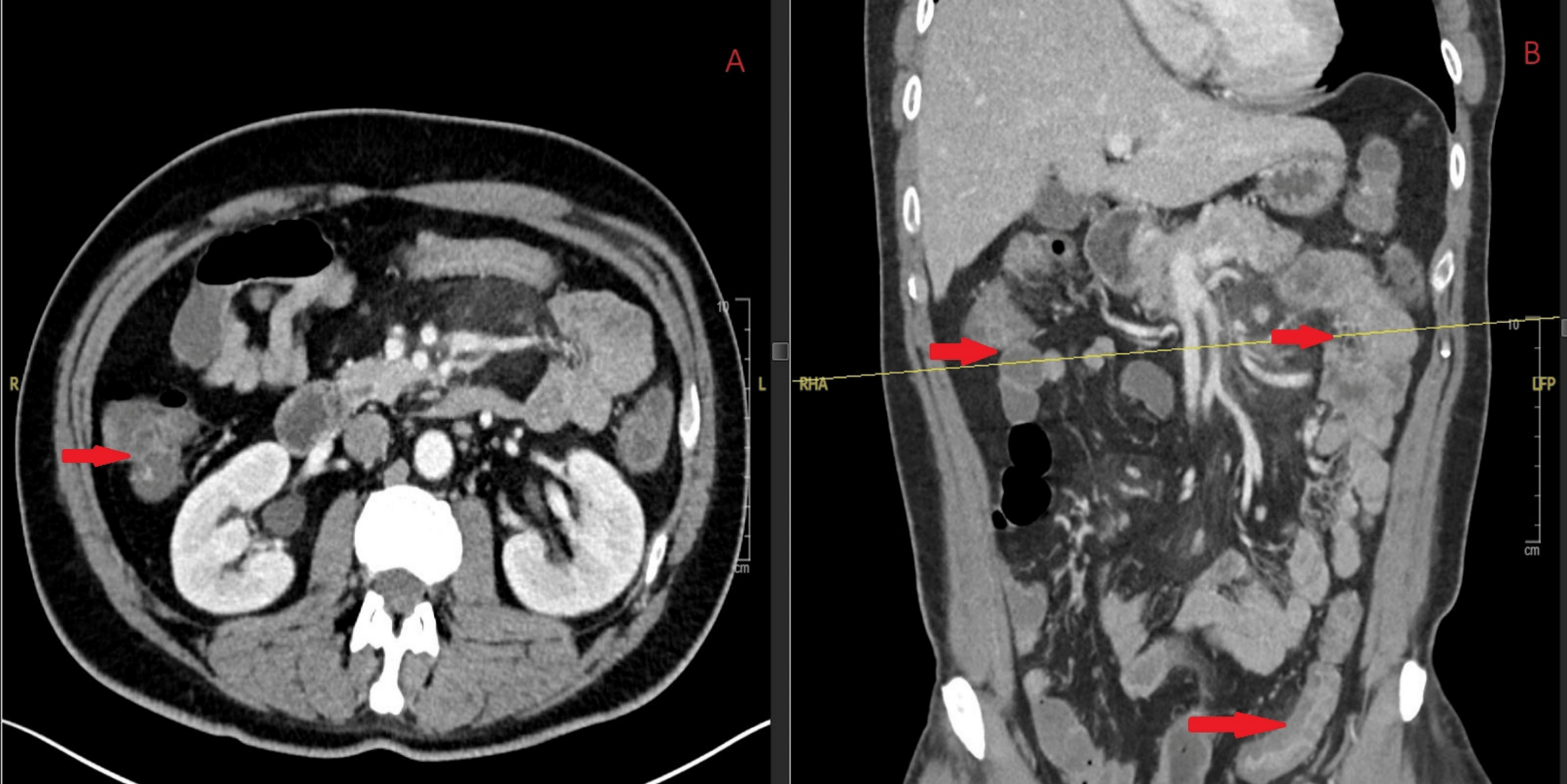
Contrast-enhanced CT of the abdomen demonstrates colonic wall thickening with pericolic fat stranding and mesenteric panniculitis (arrows). (A) Axial view. (B) Coronal view. CT: computed tomography
